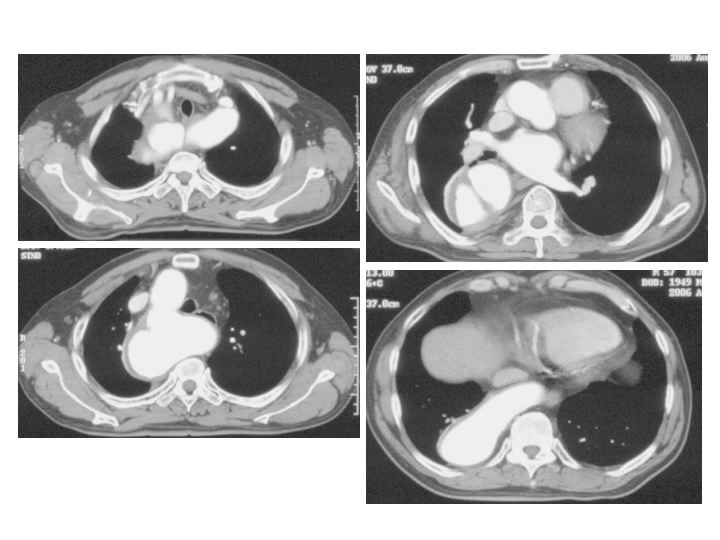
Contrast-enhanced CT scan demonstrating the dissecting aneurysm associated with the right aortic arch and left subclavian, common carotid artery from Kommerell's diverticulum.
